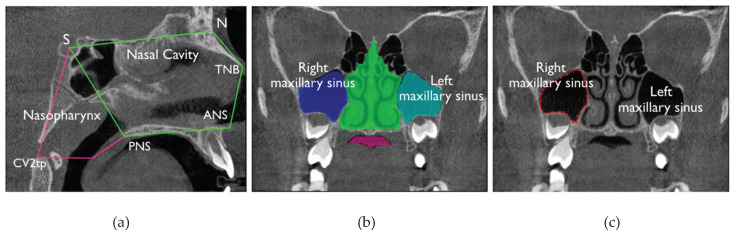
Visual explanation of planes and points used on the different cone-beam computed tomography (CBCT) axis for ITK-SNAP to perform volume segmentation of the respiratory segments analyzed: Nasal cavity, maxillary sinuses, and rhynopharynx. (a) sagittal view; (b,c) coronal views.
